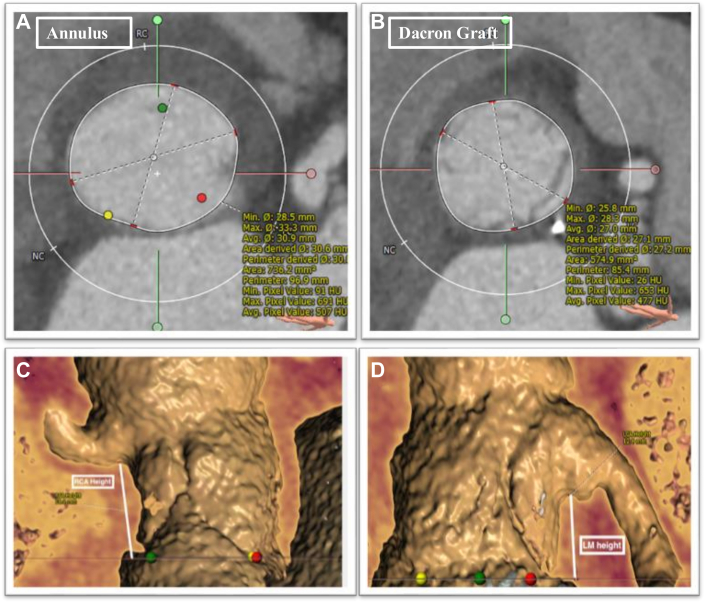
Preprocedural Gated Computed Tomography Measurements (A) Annular, (B) Dacron graft measurement, (C) right coronary artery (RCA), and (D) left main coronary artery (LM).
